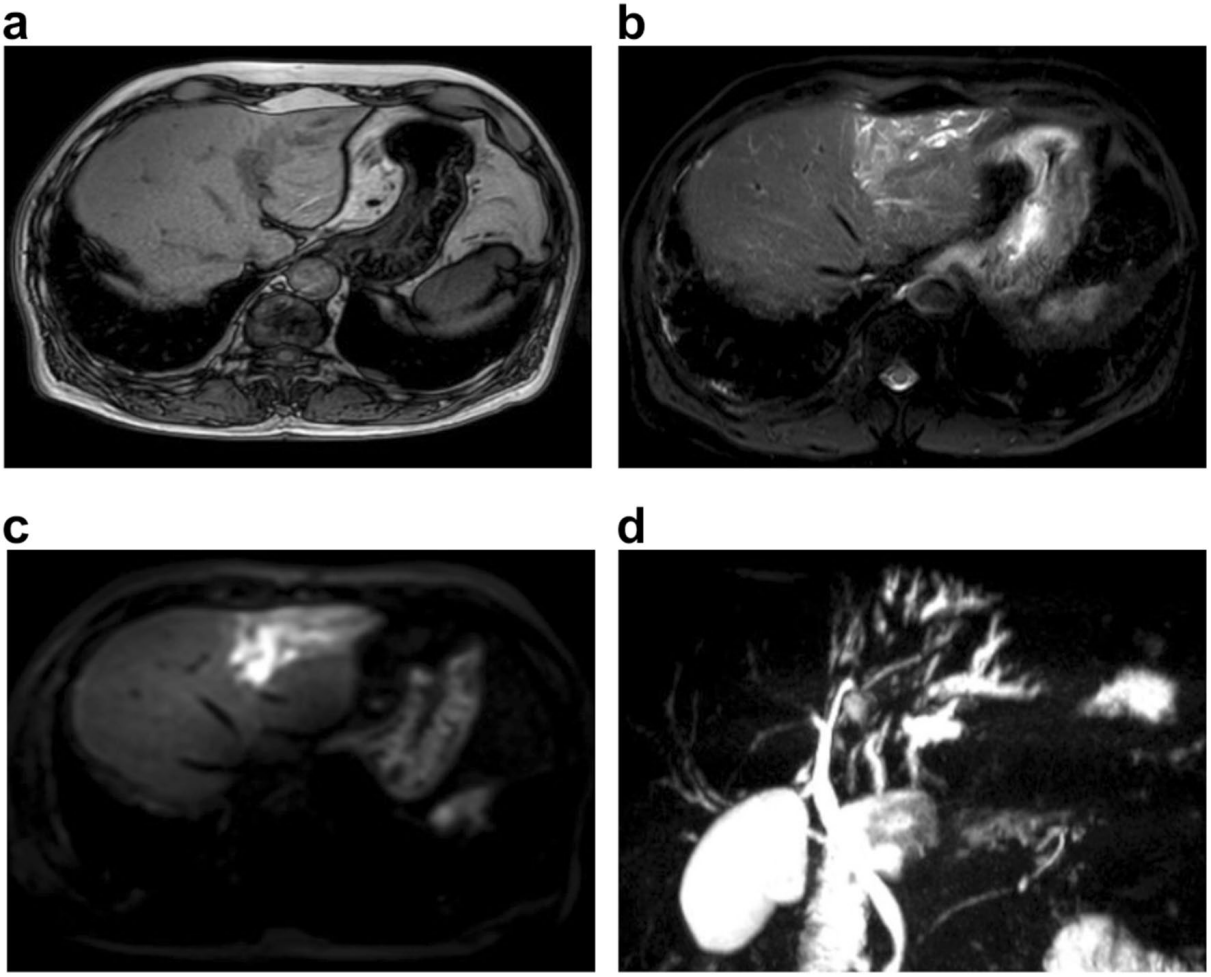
Magnetic resonance imaging scans showing a a low-intensity mass on a T1-weighted image, b a faint high-intensity mass on a T2-weighted image, a and a high-intensity mass with a low apparent diffusion coefficient value on DWI (c). Magnetic resonance cholangiopancreatography (MRCP) image showing dilatation of the peripheral bile duct
